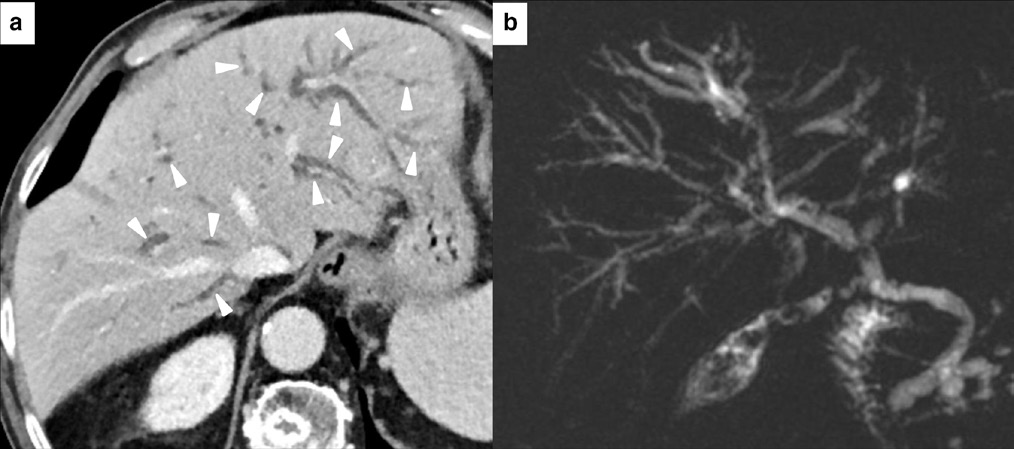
Imaging of immuno-mediated cholangitis in a 78-year-old male with metastatic melanoma, after 10 months of treatment with Atezolizumab. CT scan demonstrated bilateral intrahepatic bile duct dilatation (white arrowheads in A). Blood liver marker showed an increase of GGT, while transaminase levels were normal. MR cholangiopancreatography (B) confirmed CT findings and other causes of obstruction were not found. This pattern was classified as immuno-mediated cholangitis.
